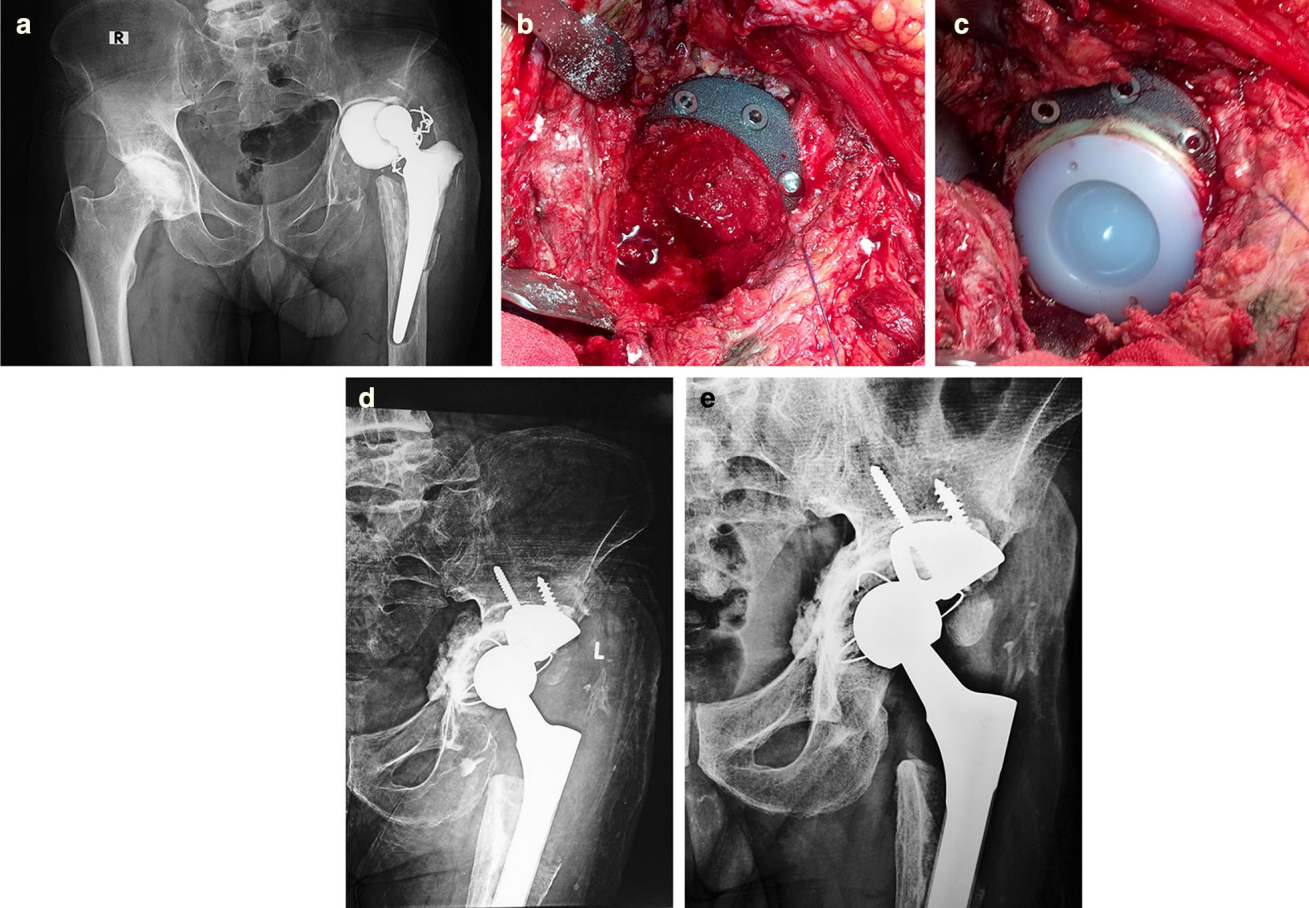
a Preoperative X-ray for a 62-year-old patient with infected hip spacer and acetabular defect grade IIIA. b Intraoperative photo showing insertion of 15-mm TMA that covers the superior acetabular defect. AB-loaded graft is impacted into the medial acetabular defect. c All-polyethylene cup is cemented into position and supported by the superior metal augment. d An X-ray 6 months postoperatively shows the cup position and bone graft. The cup is 5 mm above the hip anatomic center of rotation. e Follow up X-ray after 2 years shows maturation of the impaction graft, stable cup with no radiolucent lines, and well-integrated TMA
